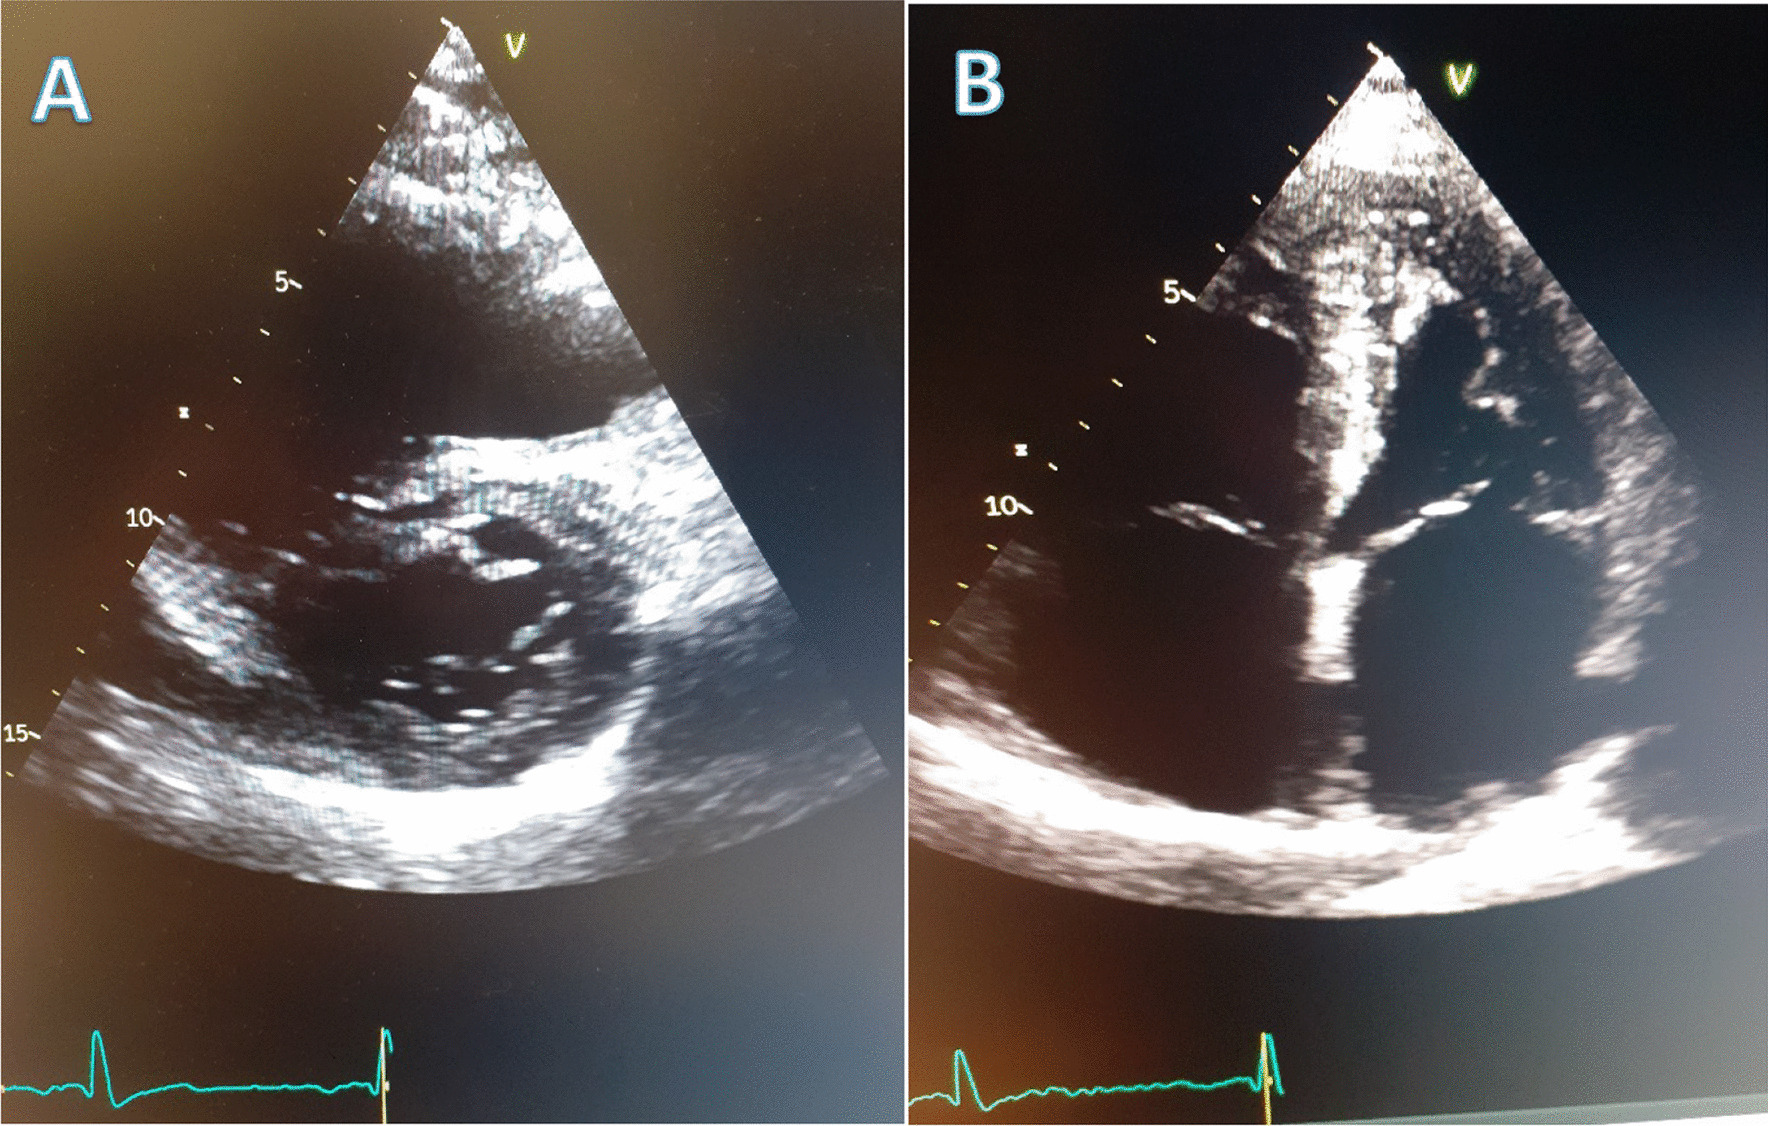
Echocardiogram evidence of right ventricular hypertrophy (A) and of right atrial enlargement (B) both consistent with underlying pulmonary arterial hypertension
